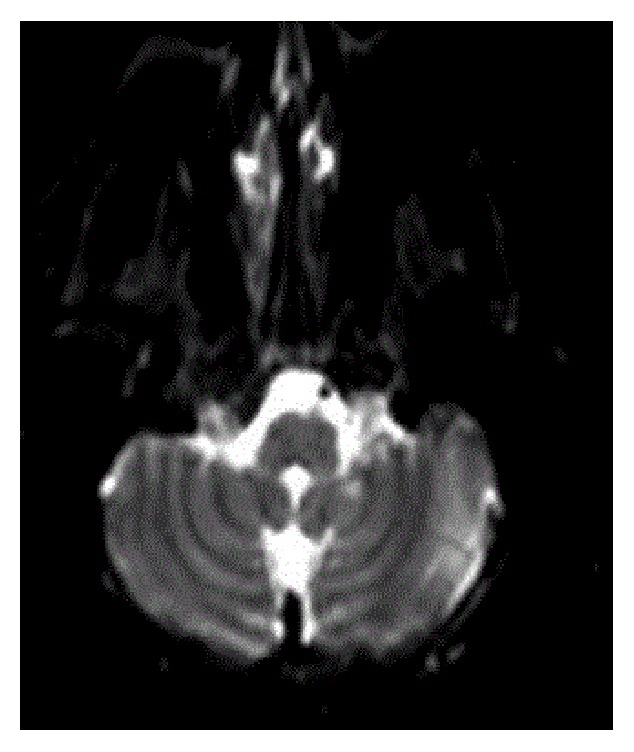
MRI of head on admission, showing multiple infarctions in the left cerebellum.
